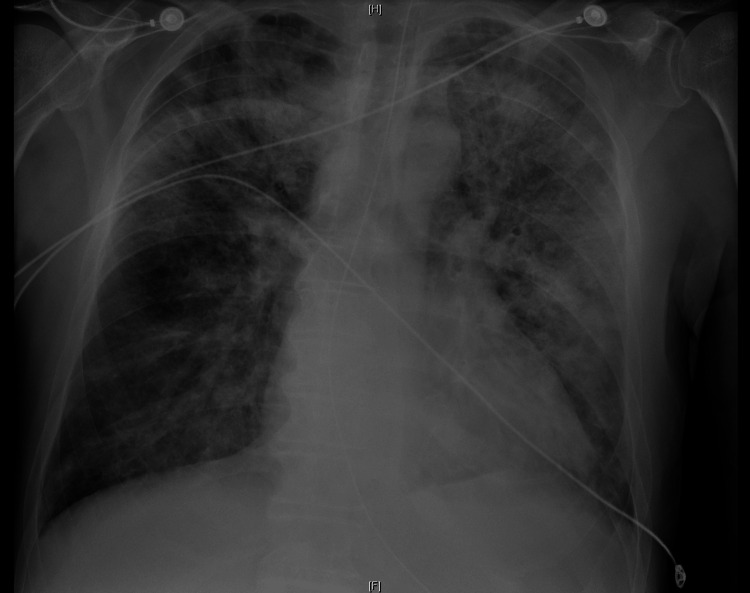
Chest X-ray evidencing bilateral pneumonia
